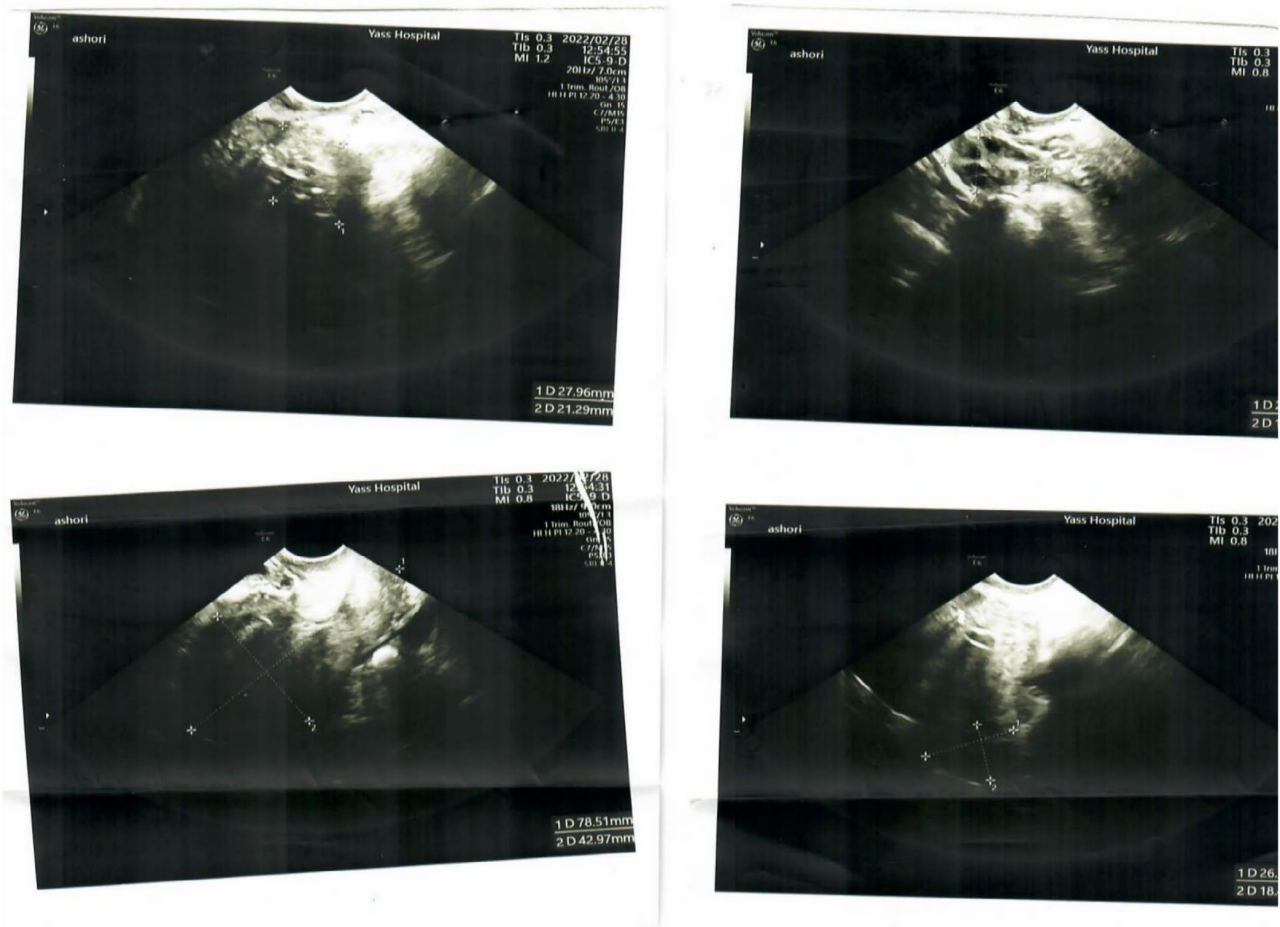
Subsequent transvaginal ultrasound revealed a heterogeneous echogenic mass (29 × 26 × 22 mm) with irregular cystic regions in the anterior portion of the uterus at the inferior part of the cornea that has high flow and low resistance vascular Doppler within the lesion. Myometrial thickness at the site of the lesion was 2 mm.
